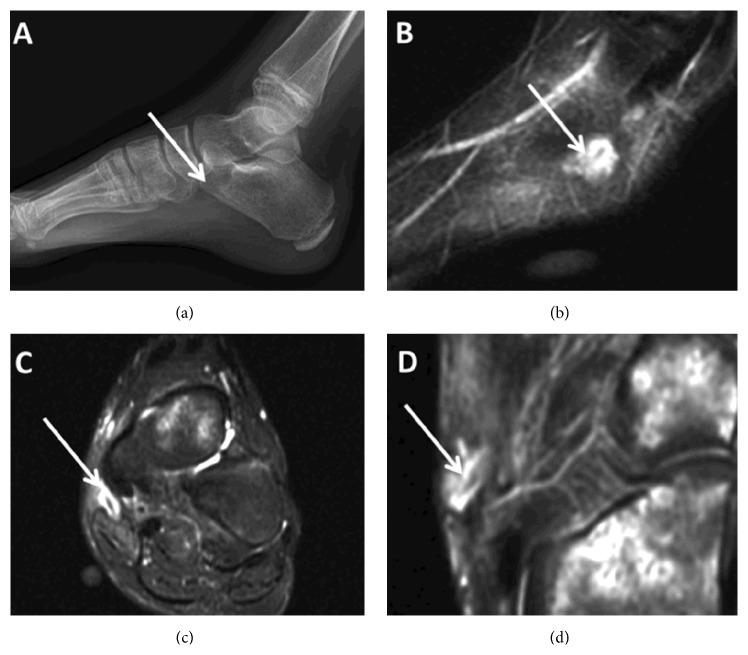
(a) X-ray and ((b)–(d)) sagittal, axial, and coronal MRI, respectively, indicating abscess with potential foreign body.
